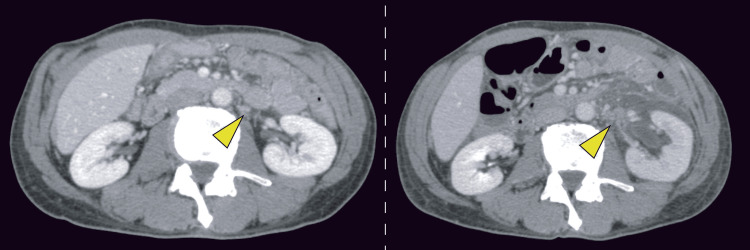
Contrast-enhanced abdominal CT CT obtained one week before admission (left panel) and on hospital day two (right panel). Yellow arrows indicate retroperitoneal metastatic lesions.
